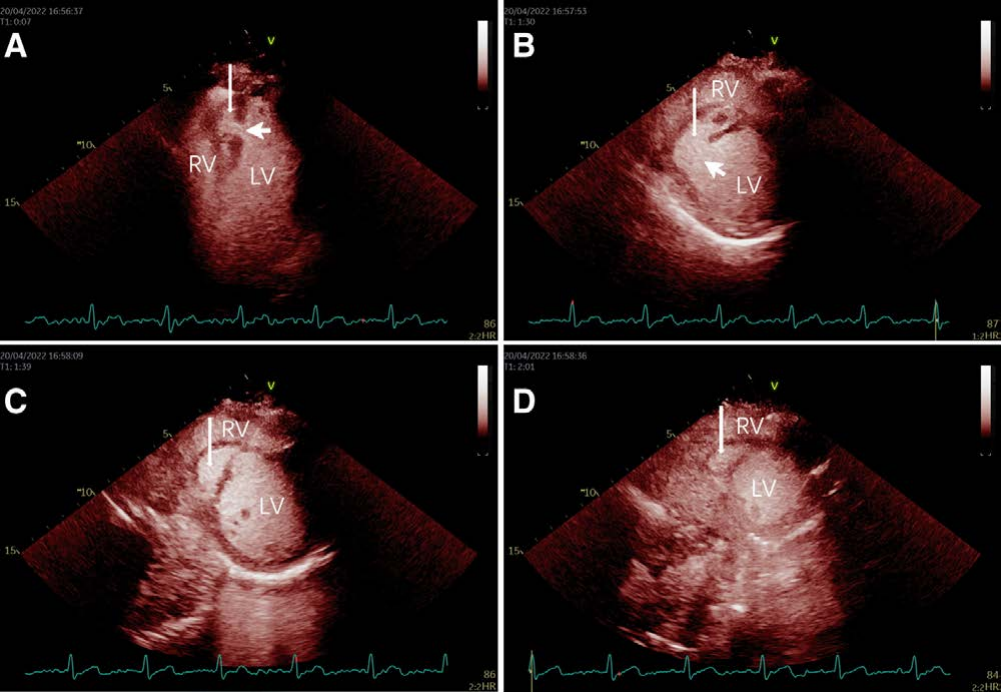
Contrast enhance-ultrasound (CEUS) imaging. The apical 4-chamber view, (A) the parasternal left ventricle short-axis view, (B) the parasternal papillary muscle short-axis view, (C) and the parasternal apical short-axis view, and (D) showed sequential visualization of the left atrium and left ventricle, the contrast agent was seen to enter the bilayer structure (long arrows) from the left ventricular cavity through the continuity interruption (short arrows) and was visualized simultaneously with the left ventricle.
